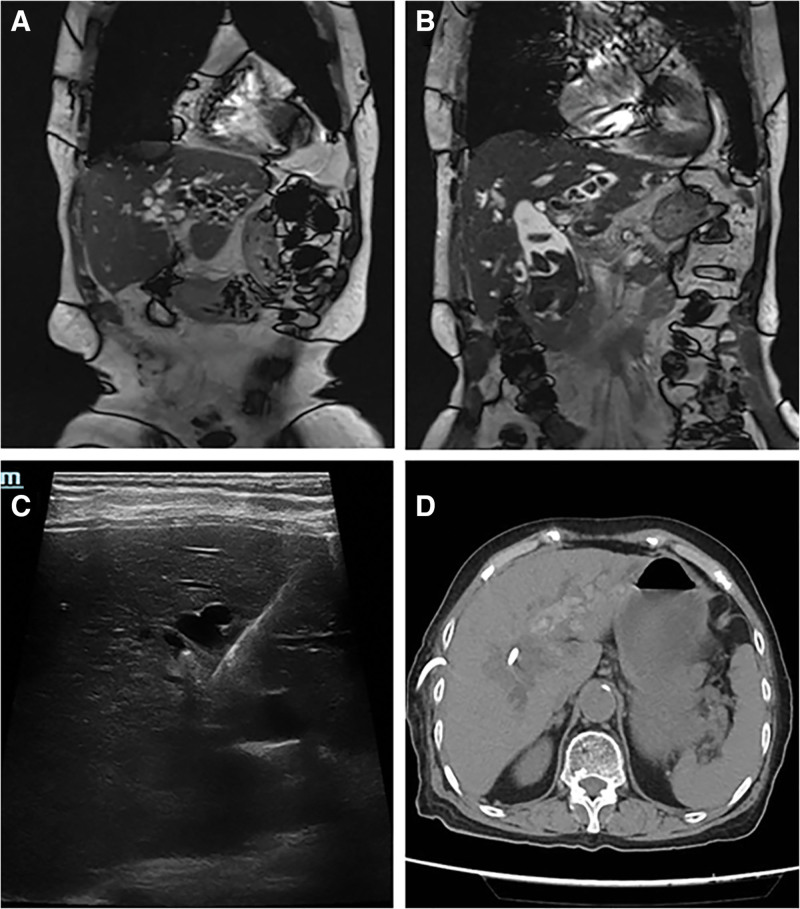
Diagnostic imaging demonstrating biliary calculus characteristics in an 86-year-old female patient (A and B). Emergency bedside ultrasound-guided drainage catheter placement (C), followed by postoperative abdominal CT verification of catheter positioning (D). CT = computed tomography.
